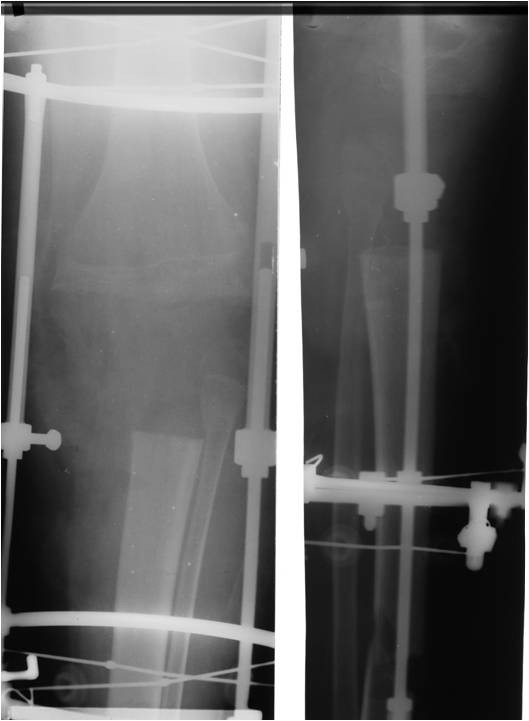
Intraoperative radiograph following en-bloc bony resection.
